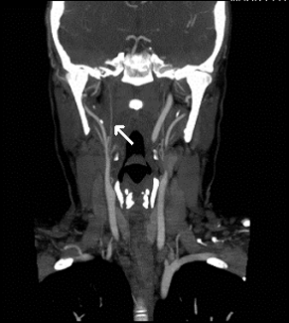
CT angiogram of carotid vessels with complete occlusion (no contrast) of the right ICA (arrow).
